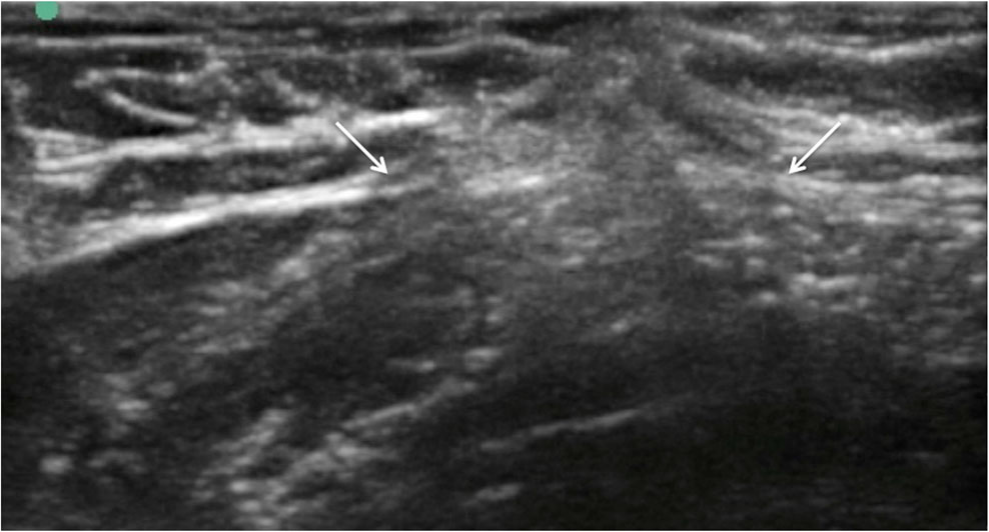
Ultrasound image of fascial layer post thread release demonstrating divided and retracted fascial edges (*white arrows*)
